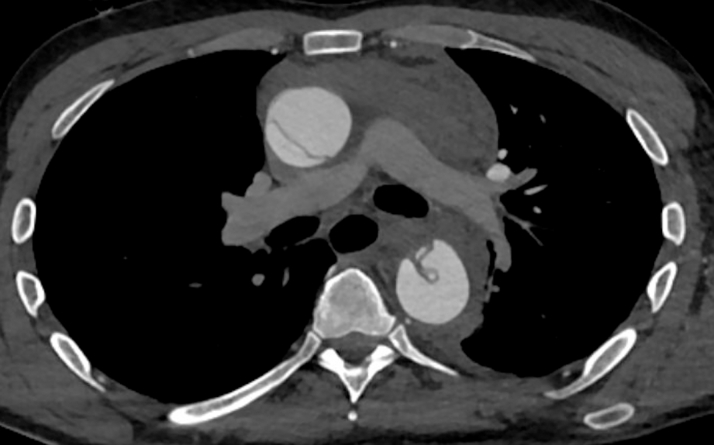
Axial Computed Tomography Demonstrating Acute Dissection of the Ascending and Descending Aorta
